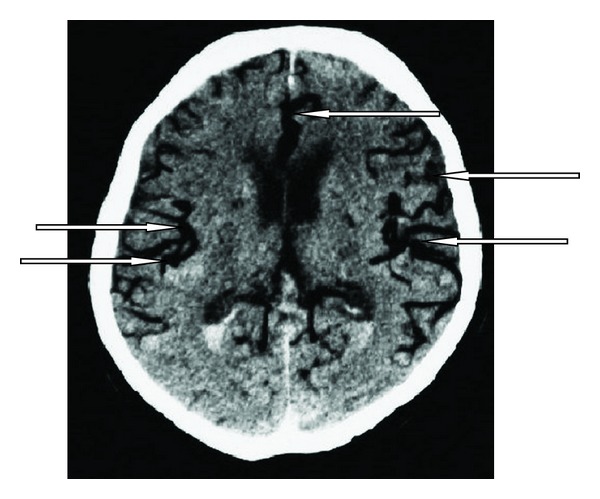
A section of cranial CT scan in the 8th day of admittance. Arrows show arterial air embolism.
